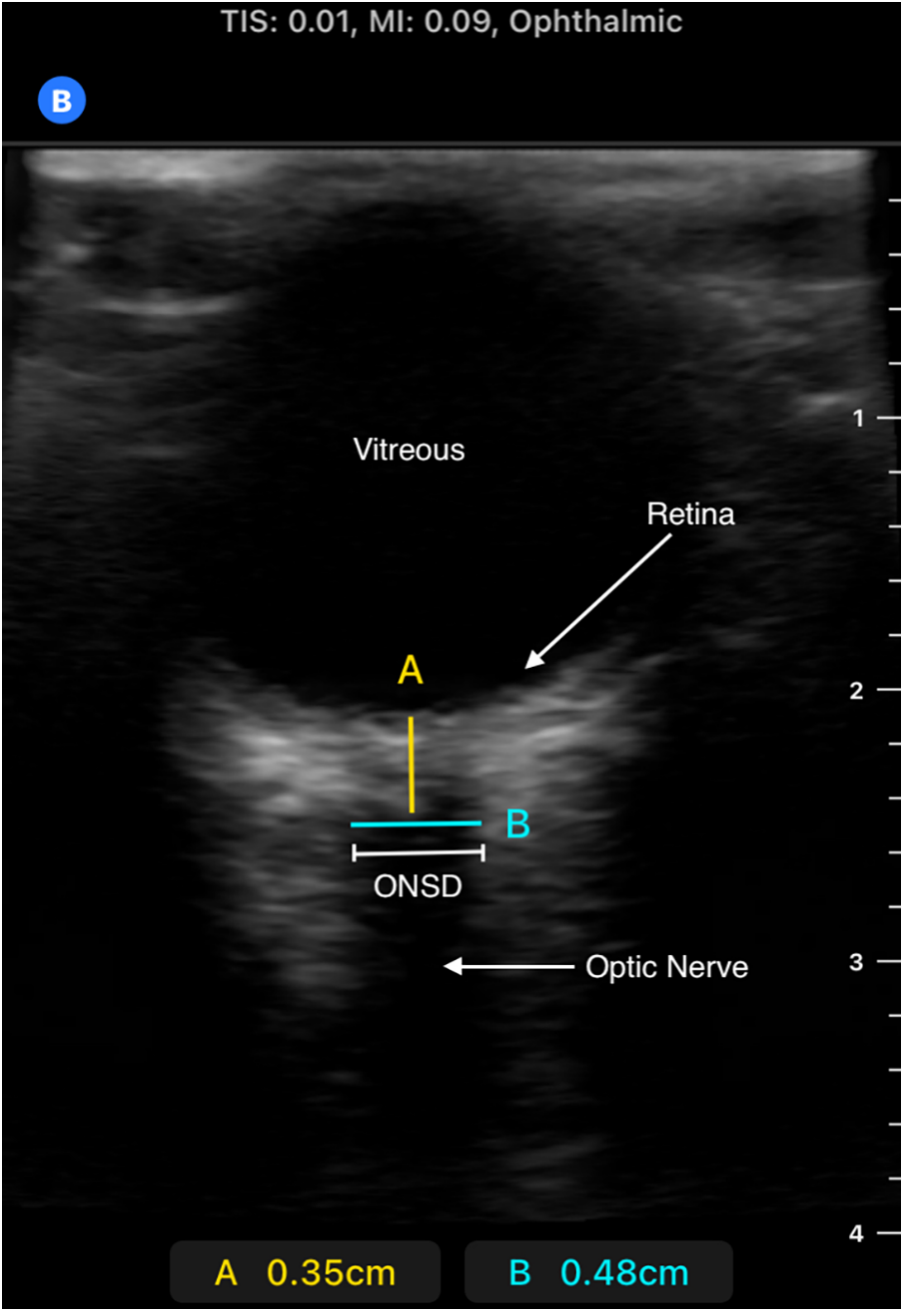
Ultrasound image of retina, optic nerve, and optic nerve sheath. Image obtained using B-mode technique; measurement made approximately 3 mm posterior to retina (measurement “A”). The anechoic “strip” in this region is the optic nerve. A hypoechoic sheath is visible immediately lateral to the optic nerve, representing the ideal location to take the ONSD measurement, represented by measurement “B”. Please note this is a representative image obtained from a pediatric patient outside of our study from a Butterfly IQ+ device which was not used in our patient population.
